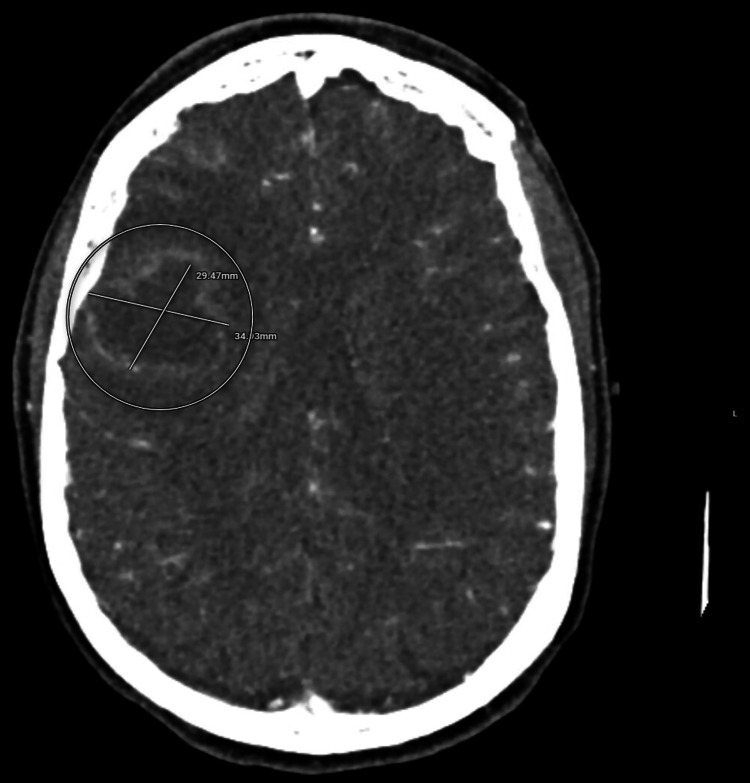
Computed tomography (CT) head with contrast, axial view, showing a 3.5 × 3.0 cm rim-enhancing mass in the right frontal region, best measured on the contrast-enhanced images.
